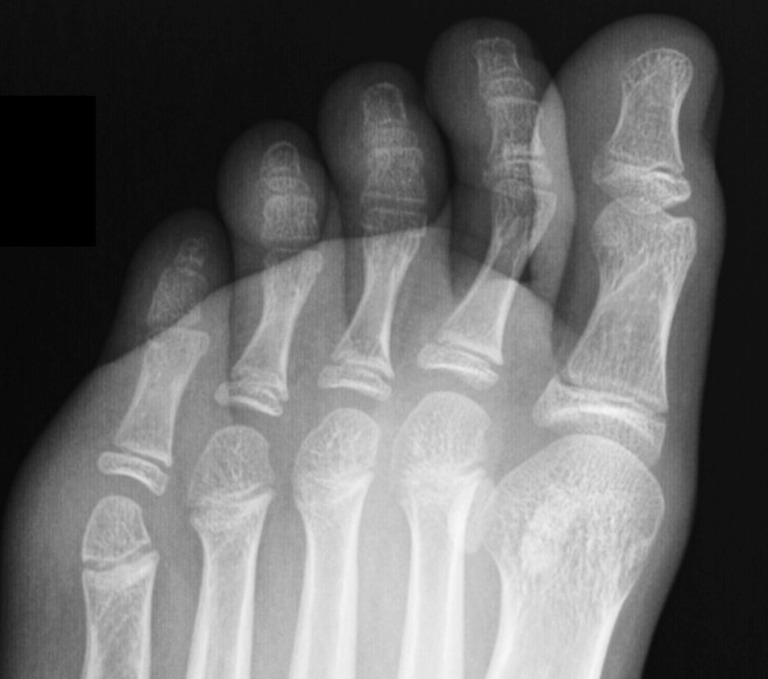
Foot radiographs of a 11-year-old female shows a 4-center 5th toe, before fusion into biphalangeal 5th toe
